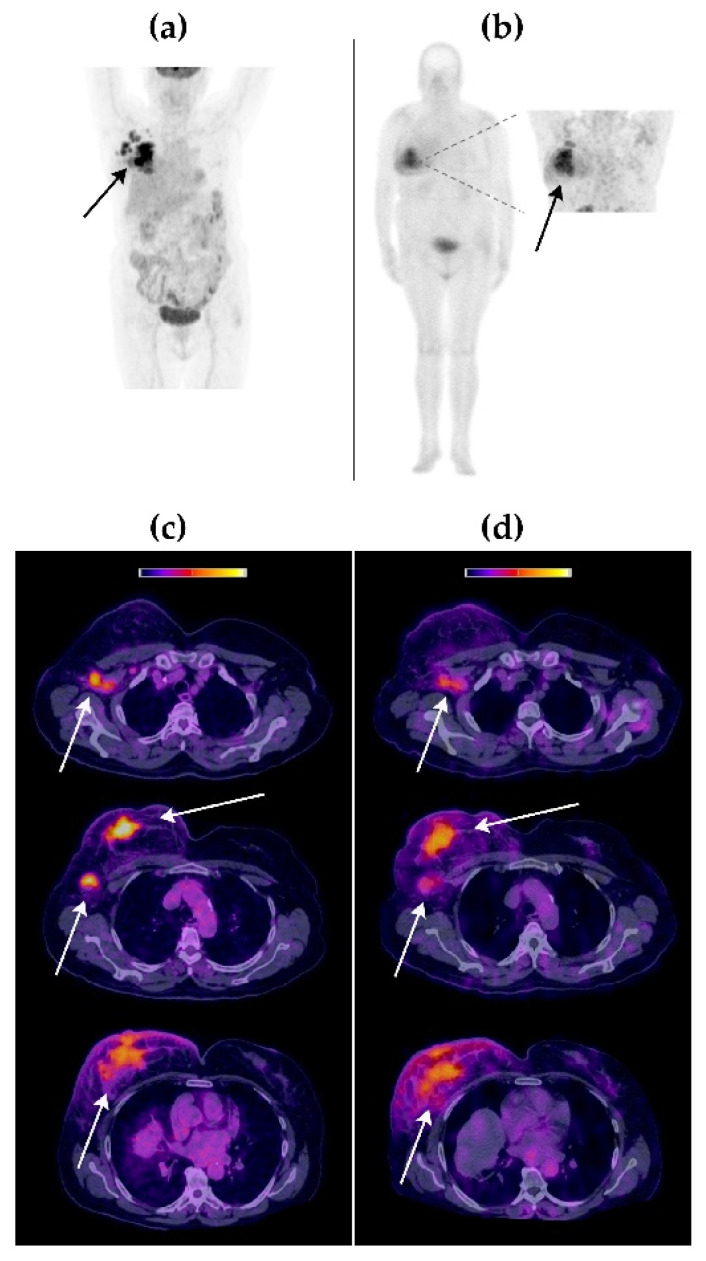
Patient with triple-negative right breast cancer (Patient 1). (a) Maximum-intensity projection [^18^F]FDG PET image at 1 h p.i. (b) [^99m^Tc]Tc-iFAP whole-body planar scintigraphy in anterior projection. Inset: thoracic SPECT at 3 h p.i. (c) [^18^F]FDG PET/CT axial sections. (d) [^99m^Tc]Tc-iFAP SPECT/CT axial sections. Right mammary gland with increased size and thickening of the generalized cutaneous plane, with a solid lesion as well as poorly defined borders in the interline topography of the upper quadrants that extends to the retroareolar region, conditioning its retraction (primary tumor). Ipsilateral axillary lymphadenopathies (Berg levels I and II). The uptake of both radiopharmaceuticals was concordant in all tumor lesions.
